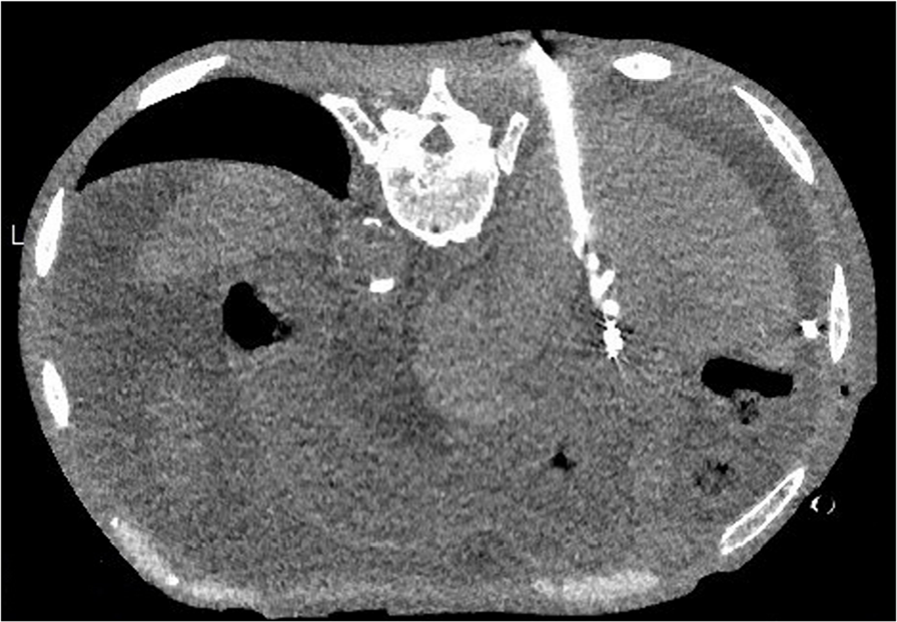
Puncture with computed tomography guidance
